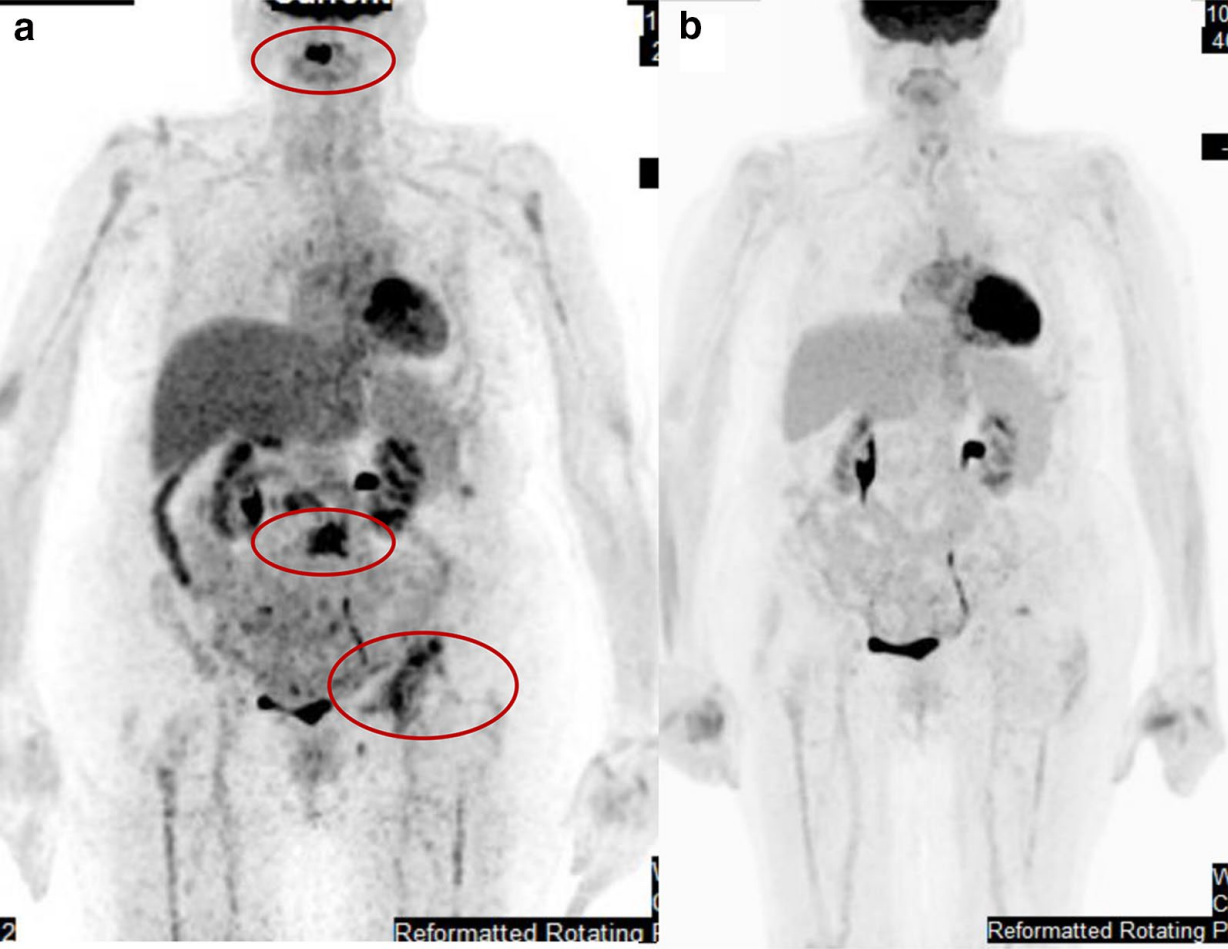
a PET scan of the patient before initiation of dabrafenib reveals metastatic disease to the left iliac bone, C2 and L3-4 vertebral bodies. The C2 lesion’s SUV max was 7; the lesion at L3 had a SUV max of 7.1; the left acetabulum lesion’s SUV max was 5.1 prior to starting dabrafenib. b After 4 months of dabrafenib therapy, near complete resolution of PET activity in the areas of bone metastases was demonstrated without any new site of disease. Upon the best response to dabrafenib achieved, the metabolic activity resolved at C2 and L3 lesions. The left acetabulum lesion only had a very small focus of residual uptake that the max SUV was not measured
